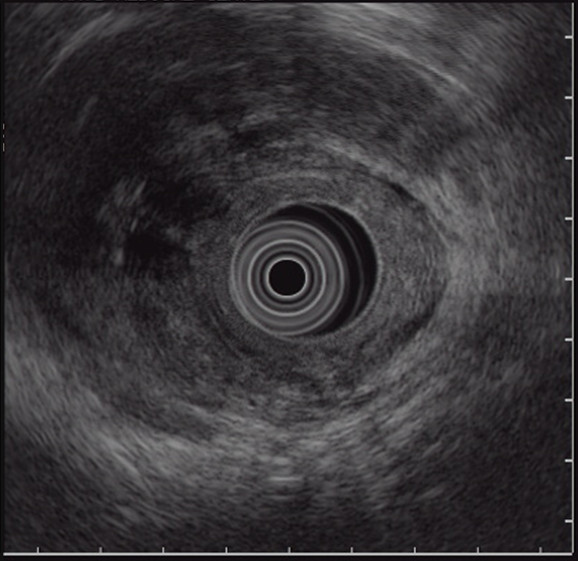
Endorectal ultrasound image showing a loculated structure, with mobile fluid, solid debris, and significant surrounding inflammation, around the rectosigmoid area.
